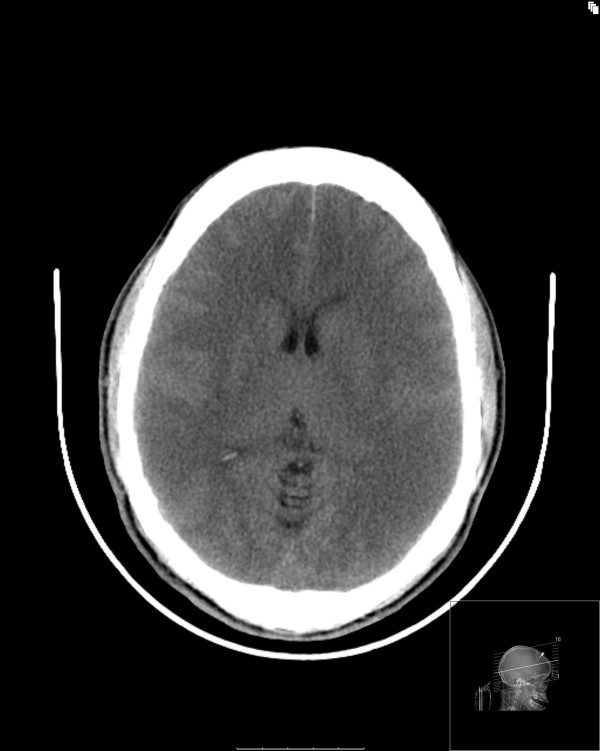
Computed tomography image showing significant edema predominantly in the supratentorial region.
